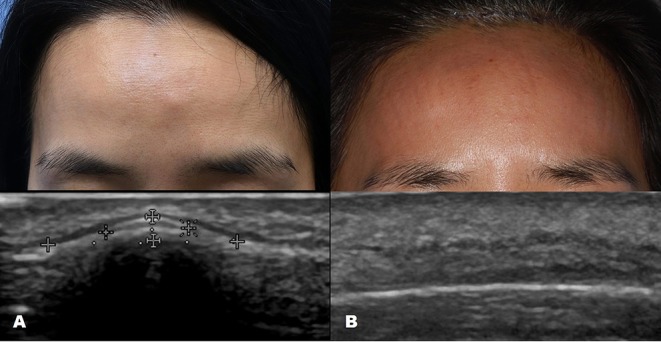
Ultrasound images of forehead osteoma. (A) Before excision. (B) After excision performed through a remote incision above the hairline.
